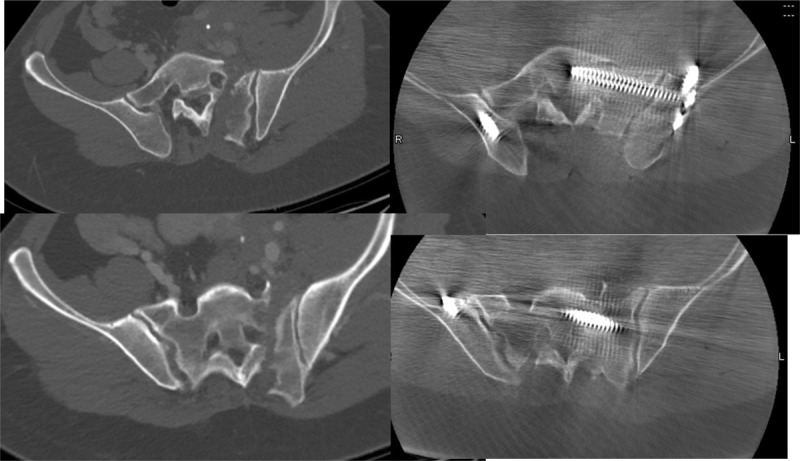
The preoperative CT scans (2 left panels) compared with the intraoperative 3D fluoroscopic scans (2 right panels) demonstrating improved albeit imperfect reduction with adequate implant position.
